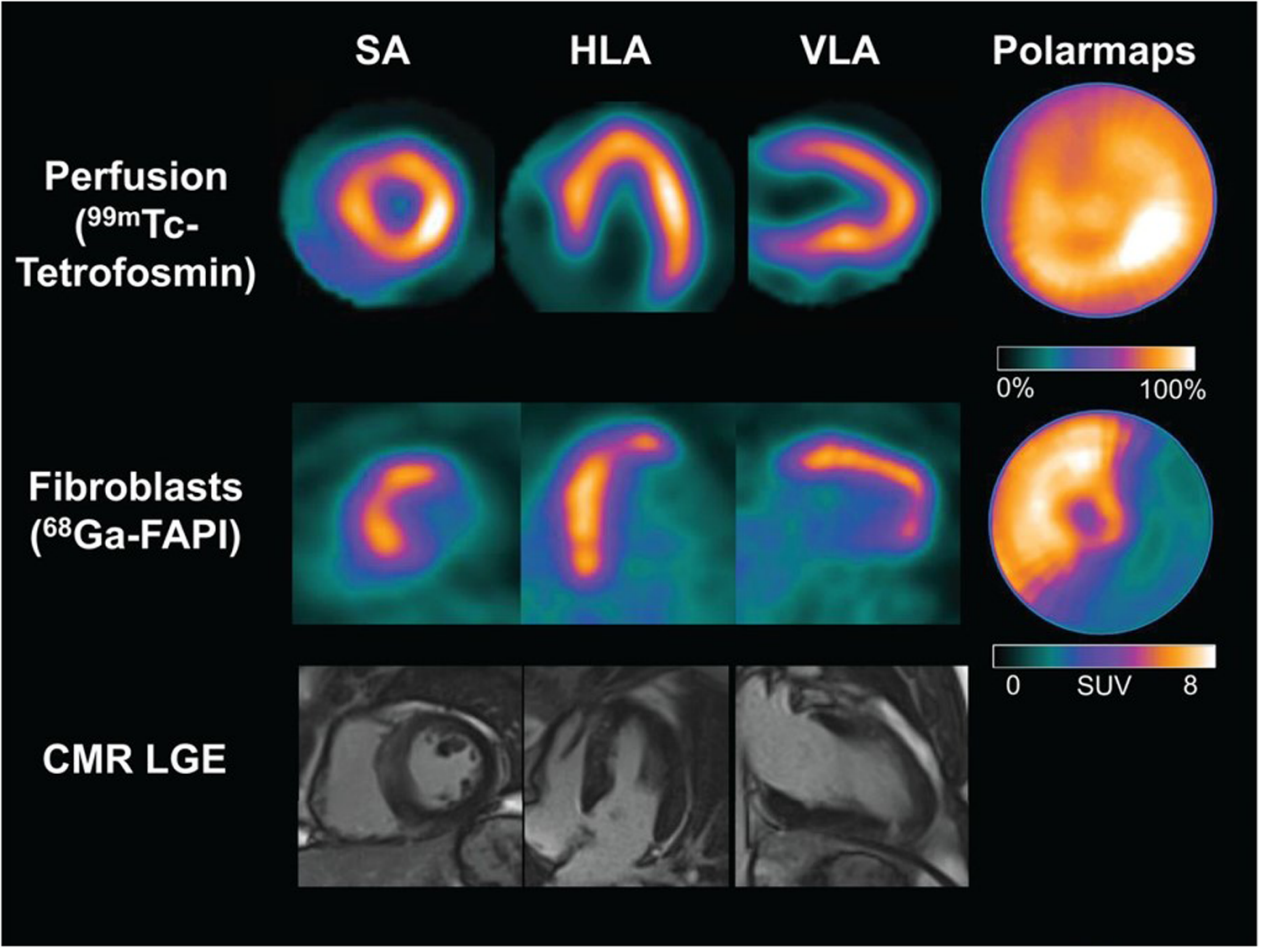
Cardiac images acquired after reperfusion therapy for acute myocardial infarction. The first row depicts single photon emission computed tomography (SPECT) myocardial perfusion images acquired at rest using [^99m^Tc]Tc-tetrofosmin, followed by [^68^Ga]Ga-FAPI-04 PET images in the second row. The third row depicts cardiovascular magnetic resonance imaging. There are no perfusion defects visualised after reperfusion therapy in the SPECT resting perfusion images. However, a large area of cardiac fibroblast activation is visualised in the anterior and septal walls and partially in the apex. There is no evidence of late gadolinium enhancement in the corresponding myocardial images on CMR. HLA, horizontal long axis; SA, short axis; VLA, vertical long axis. This research was originally published in *JNM*. Johanna Diekmann et al. (18). © SNMMI.
